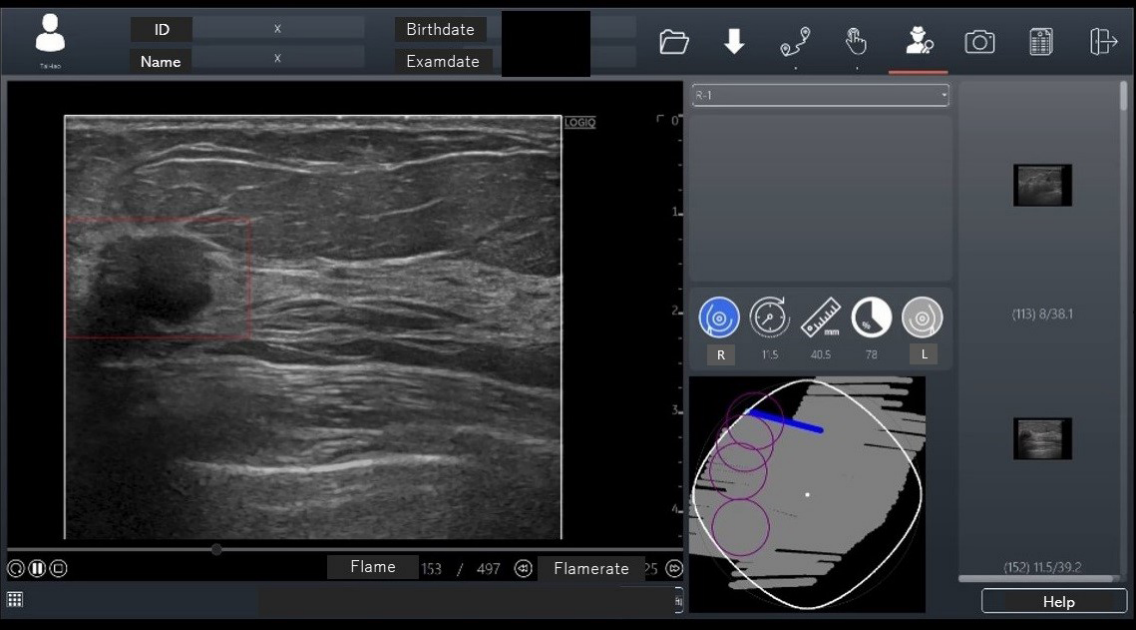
Detection of Breast Lesions Using CADe in Ultrasound In November 2020, a breast cancer detection support program was approved for the ultrasound modality in Japan for the first time. Artificial intelligence (AI) has two functions: it performs shape analysis during the examination, displaying suspected breast cancer areas as regions of interest on the image screen. The probe position is also shown on a route map screen.
